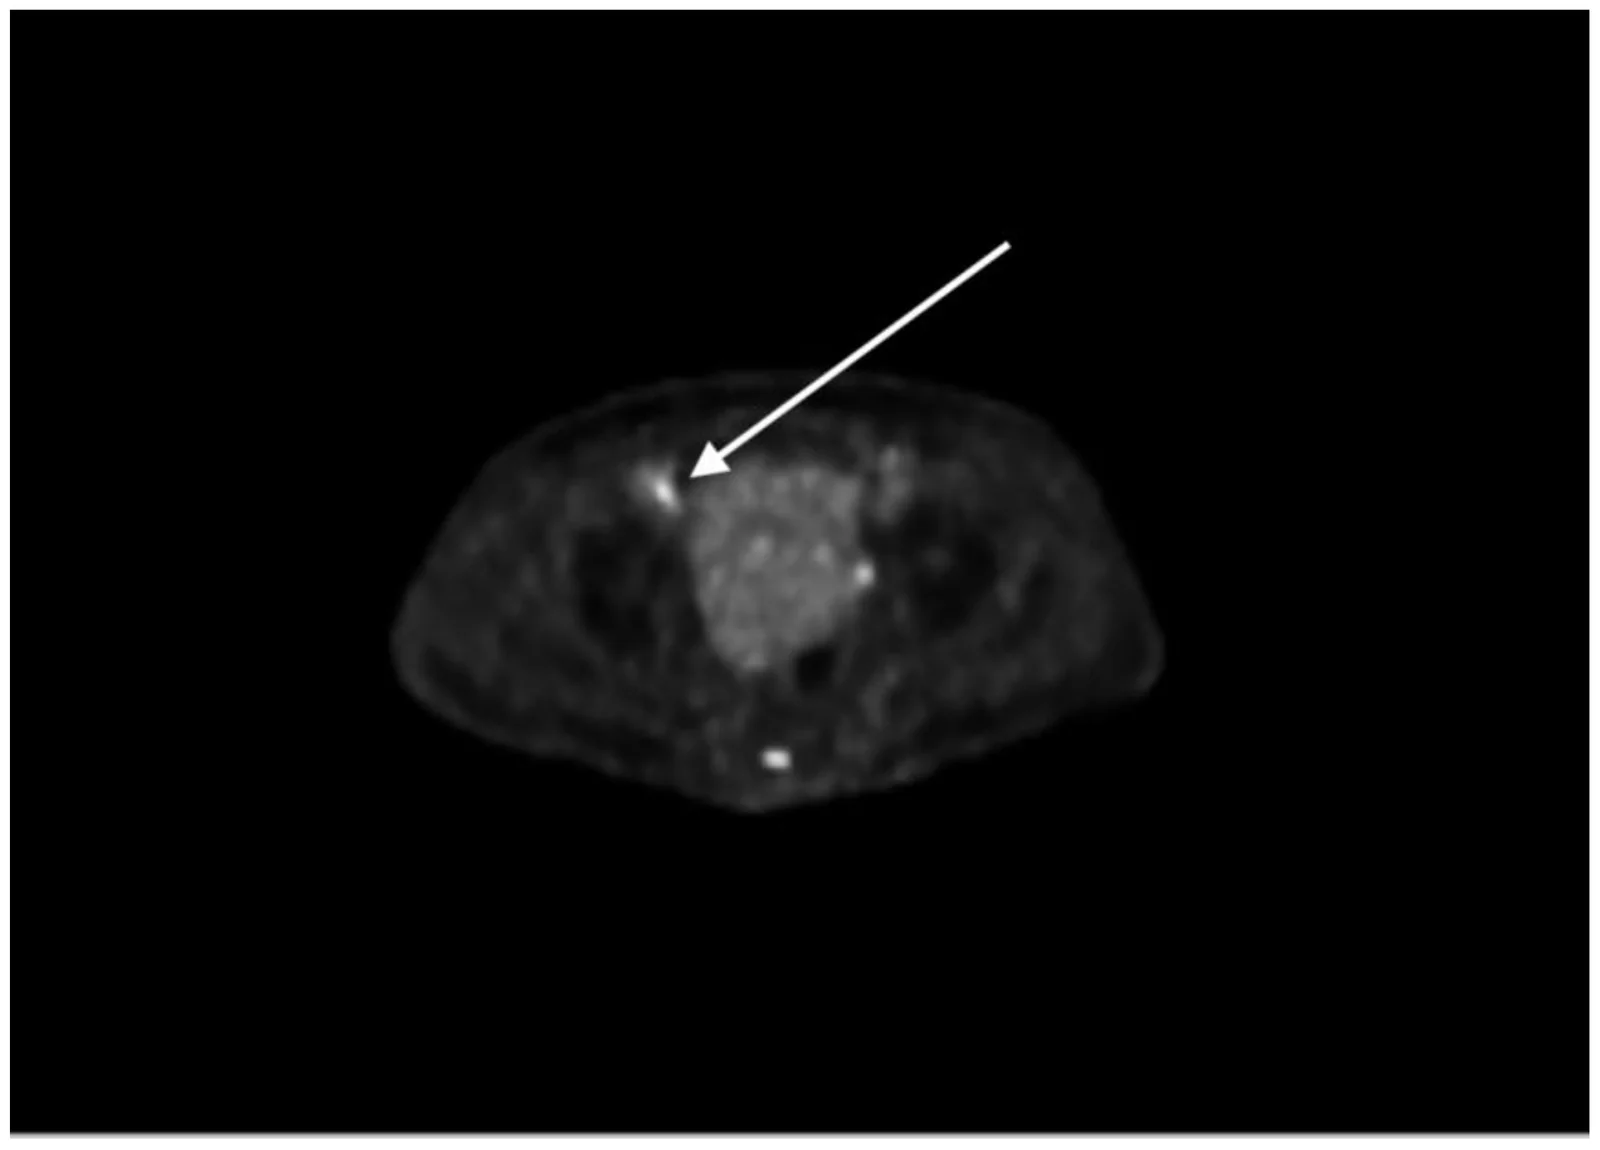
PET/CT imaging showing extensive pelvic and iliac lymph node involvement (arrow), consistent with metastatic disease.
